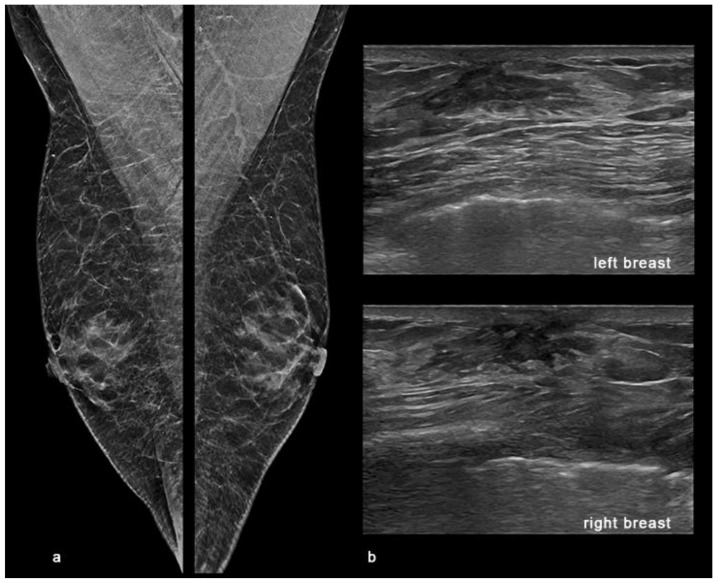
Gynaecomastia. Digital breast tomosynthesis (DBT) and ultrasound (US) showing bilateral gynaecomastia in a 74-year-old male observed at our department with tender lumps in both breasts and mastodynia at clinical examination. (a) The synthetic 2D reconstructed medio-lateral-oblique images (s2D-MLO) showed a symmetric increased flame-shaped sub-areolar density in both breasts. (b) US showed a retro-areolar, triangular, hypoechoic mass in both breasts.
